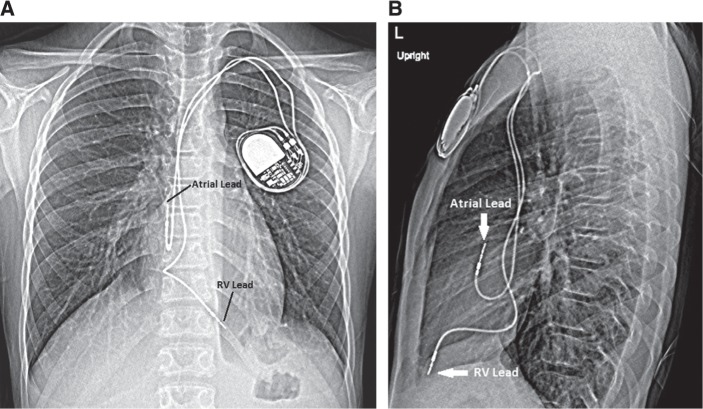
A: Anteroposterior chest radiograph showing lead placement in a dual-chamber transvenous pacing system in a structurally normal heart. B: Lateral chest radiograph showing lead placement in a dual-chamber transvenous pacing system in a structurally normal heart.
